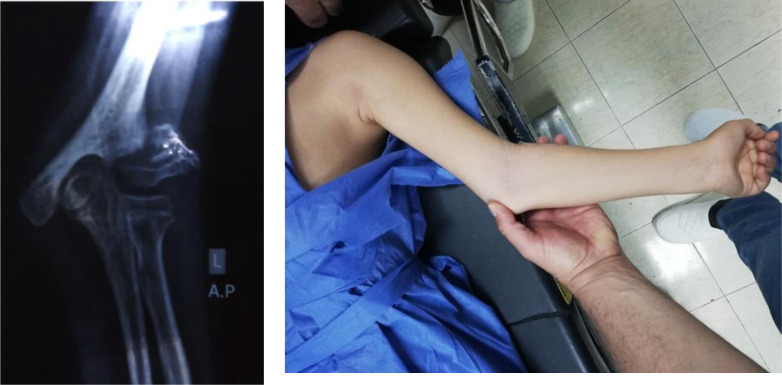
Preoperative X-ray and clinical photo of case no. (1).
